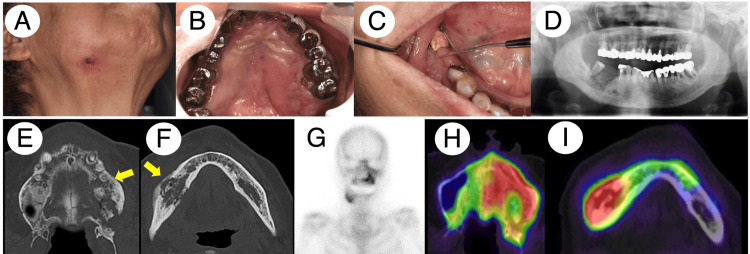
Patient 1 clinical and radiographic findings before the treatment. Extraoral examination identified a fistula in the right-sided mandibular skin (A). Intraoral examination detected bone exposure probing through intraoral fistula in the left-sided maxillary molar area and right-sided mandibular molar area (B and C). Panoramic X-ray and MDCT depicted sequestrum and abnormal sclerotic changes in the affected areas with apparent periosteal reaction in the mandibular bone (the yellow arrow indicates the signs of sequestrum in the maxillary and mandibular bones) (E and F). Bone scintigraphy and SPECT showed the increased accumulation of 99mTc-MDP/HMDP in the affected areas (G, H, and I). MDCT, multidetector computed tomography; SPECT, single-photon emission computed tomography; 99mTc, technetium-99m; MDP, methylene diphosphonate; HMDP, hydroxy-methylene diphosphonate
